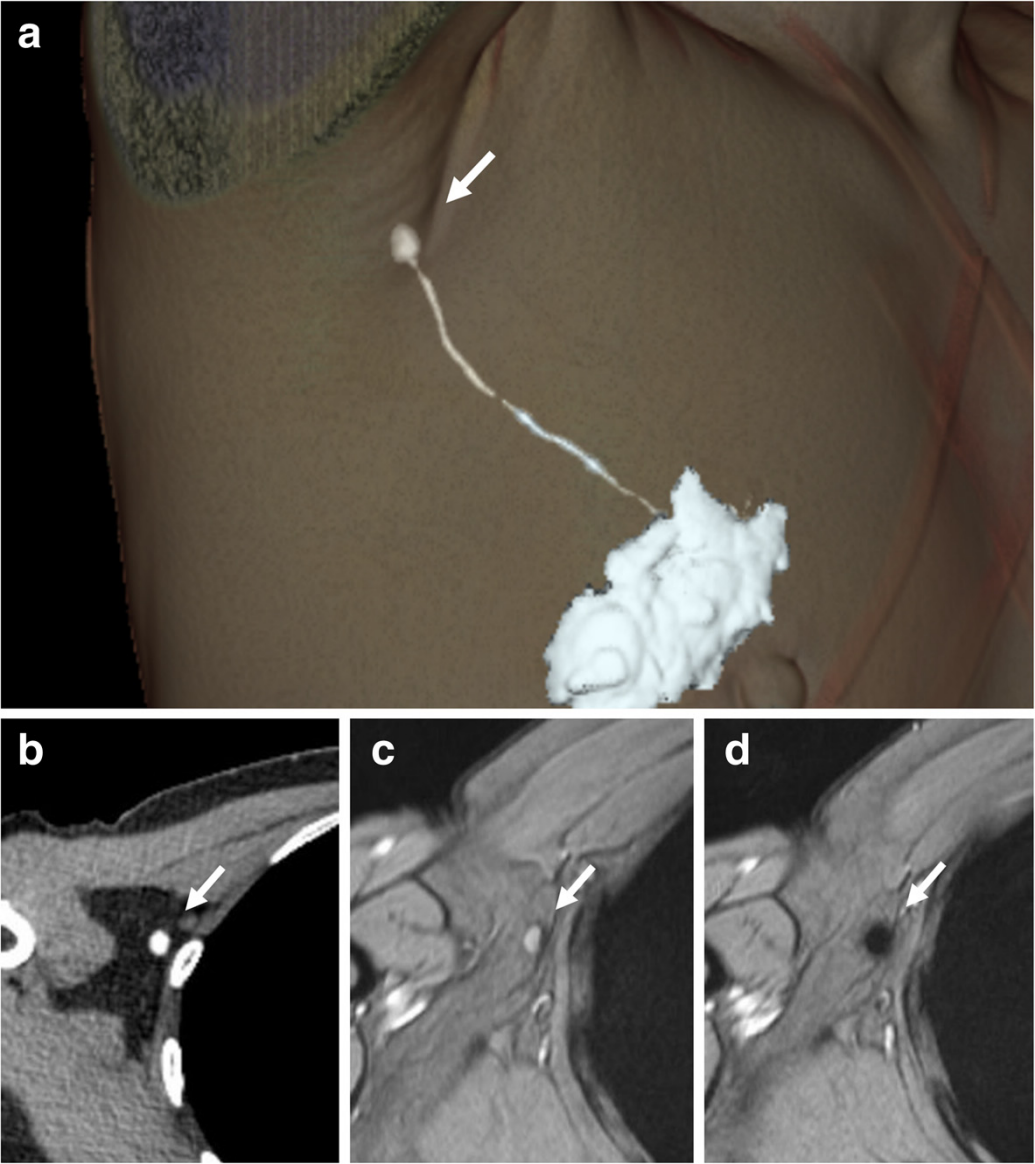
Sentinel node localization using CT-lymphography and SPIO-enhanced MR imaging for diagnosis. Three-dimensional CT-lymphography reconstructed from the first post-contrast images (a). Lymphatic vessels drained into a single axillary sentinel node (arrow). Images of CT-lymphography (b) and T2*-weighted axial MR images (c) at the same level were compared to specify the node (arrow) on T2*-weighted axial MR imaging corresponding to the sentinel node (arrow) identified by CT-lymphography. The node (arrow) showed high-signal intensity before administration of superparamagnetic iron oxide (SPIO). (d) After administration of SPIO, the node showed homogenous low signal intensity and was diagnosed as benign (arrow).
